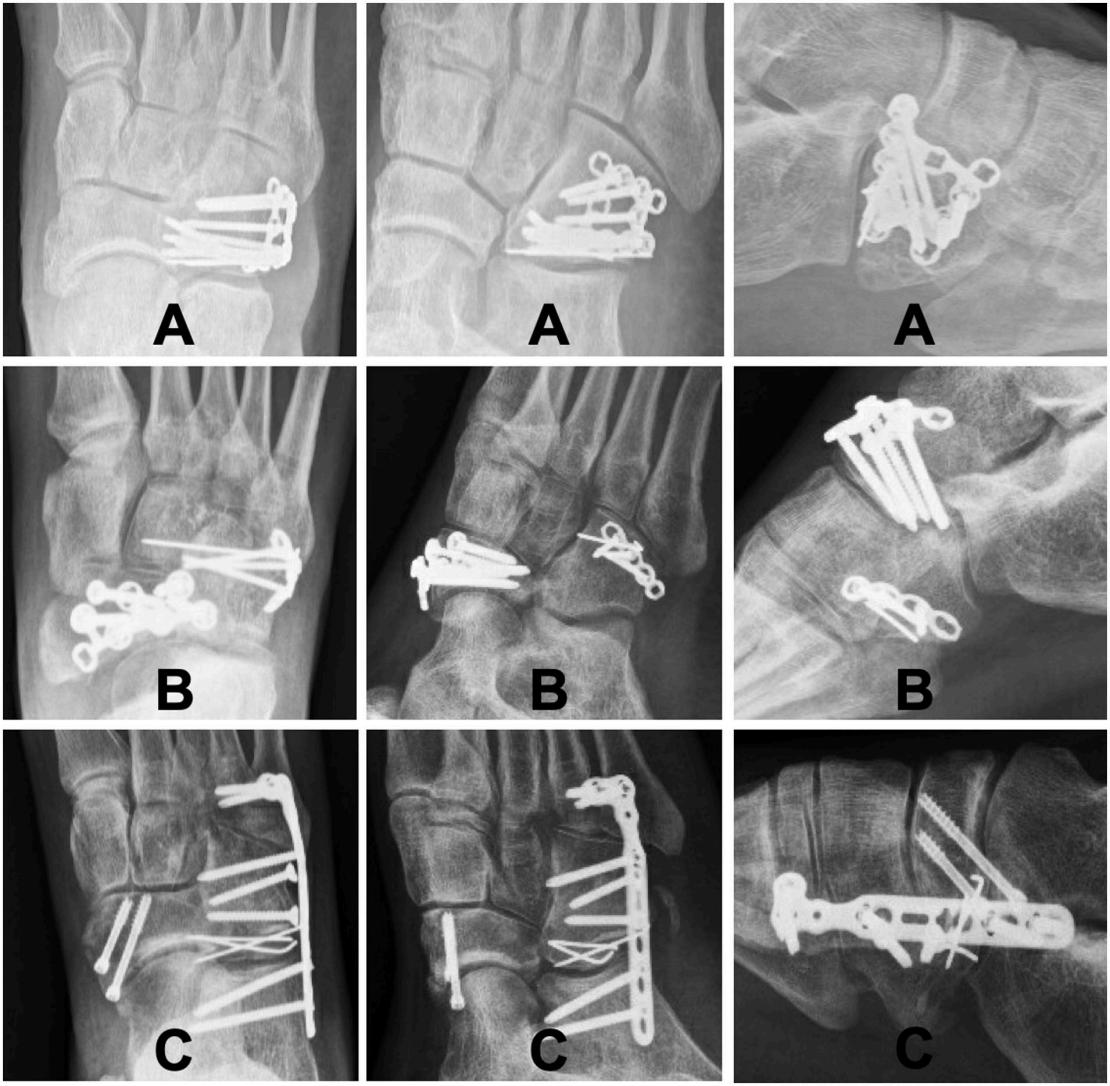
Examples of fixation techniques used. Left to right: anteroposterior, oblique, and lateral radiographs. Top to bottom: (A) ORIF cuboid with VA locking cuboid plate 2.4/2.7, (B) ORIF cuboid with 2.0 plate and 1.0 K-wire for additional fixation of small fragment, ipsilateral navicular fracture, (C) ORIF cuboid with locking bridge-plate (calcaneus, cuboid, metatarsals 4 and 5), 2x 2.7 cortical screws, 1.0 K-wires, as part of Chopart fracture-dislocation including navicular fracture.
